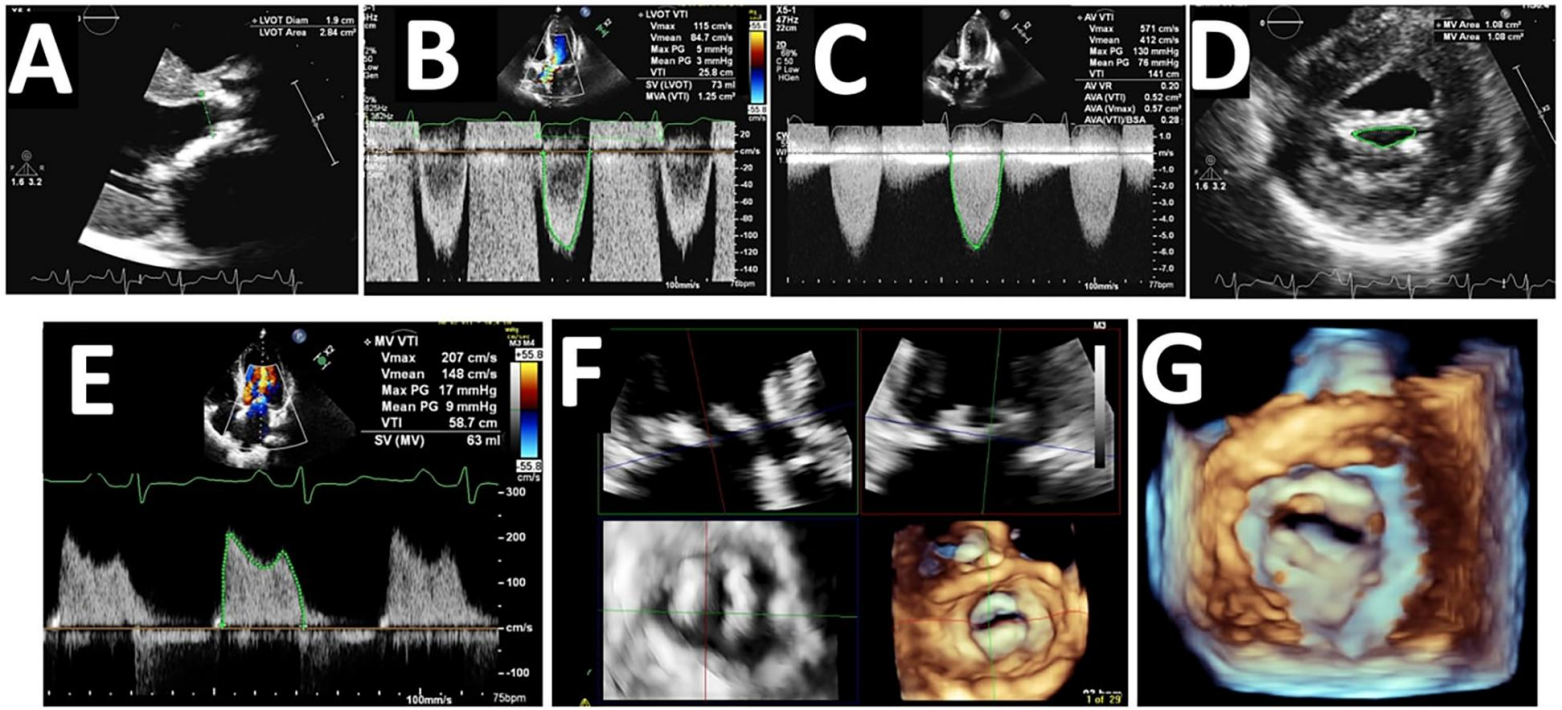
Echocardiographic findings in a patient with concomitant aortic stenosis and mitral stenosis. (A) Parasternal long-axis view showing the left ventricular outflow tract (LVOT) diameter (1.9 cm) and area (2.84 cm^2^); (B) Pulse-wave Doppler tracing at the LVOT showing velocity-time integral (VTI) of 25.8 cm and calculated stroke volume (SV) of 73 ml, with a mitral valve area (MVA) of 1.25 cm^2^ derived from VTI; (C) Continuous-wave Doppler tracing across the aortic valve showing a peak velocity of 571 cm/s and mean gradient of 76 mmHg, with a calculated aortic valve area of 0.57 cm^2^, indicating severe AS; (D) 2D parasternal short-axis view of the mitral valve showing MVA by planimetry (1.08 cm^2^), consistent with severe MS; (E) Continuous-wave Doppler of the mitral valve showing peak velocity of 207 cm/s and mean transmitral gradient of 9 mmHg, further confirming MS severity; (F) 3D echocardiographic multiplanar reconstruction of the mitral valve, allowing detailed anatomical evaluation of leaflet fusion and valve morphology; (G): 3D-rendered image of the mitral valve orifice, showing the restricted valve opening and commissural fusion in severe MS.
